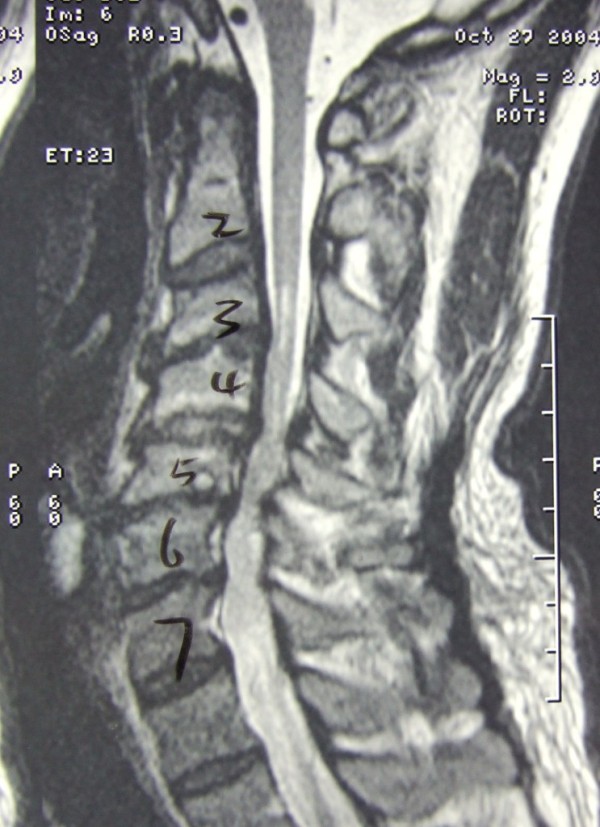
High-signal intensity area in the C3/4 segment in T2-weighted MRI image.
